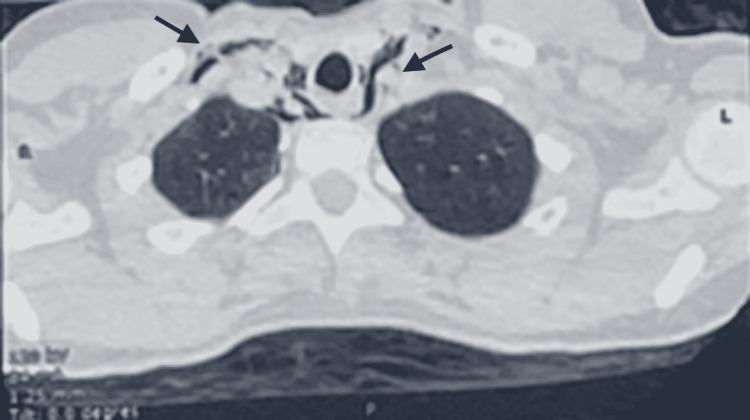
Thoracic CT scan images showing pneumomediastinum and cervical subcutaneous emphysema.
